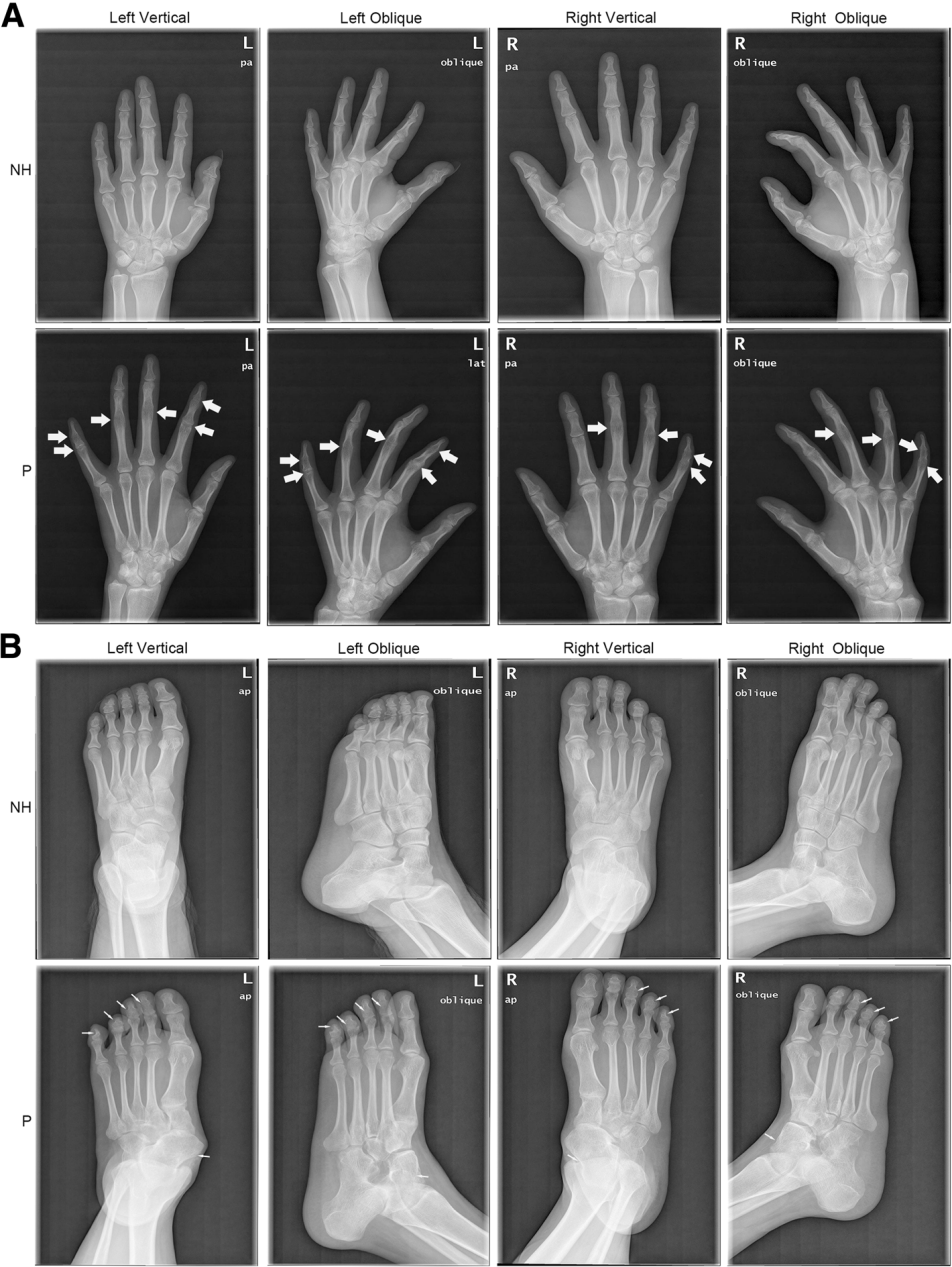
Radiographs of hands and feet of the proband and her normal mother. a Vertical and oblique views of both hands are shown. Arrows indicate interphalangeal-joint fusion in the digits. b Vertical and oblique views of both feet are shown. Arrows indicate interphalangeal-joint fusion in the digits and talonavicular synostosis
